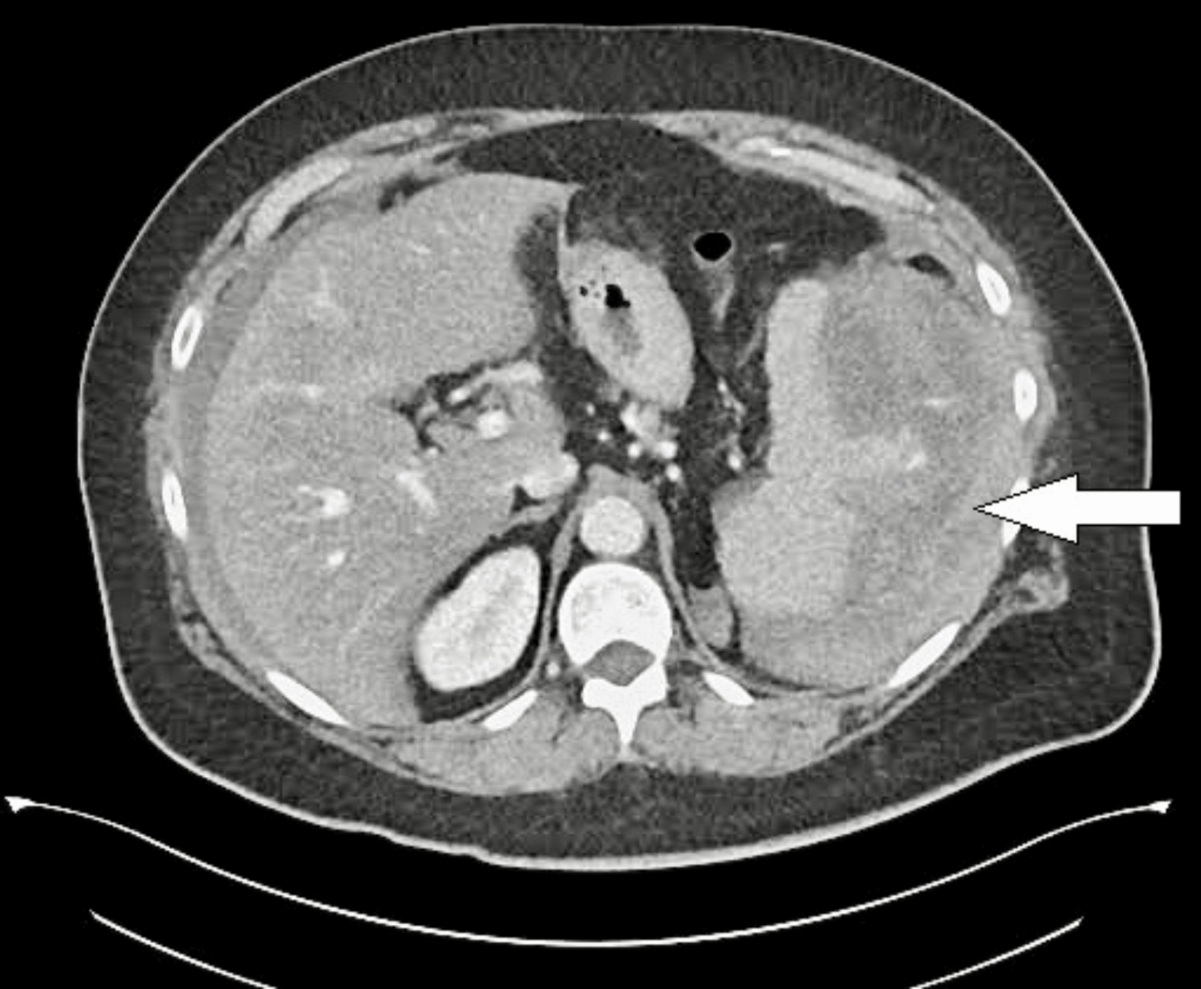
Congenital afibrinogenemia with spontaneous splenic hemorrhage Contrast enhanced CT abdomen and pelvis showing moderate hemoperitoneum with splenic parenchymal rupture. The white arrow indicates the splenic parenchymal rupture.
